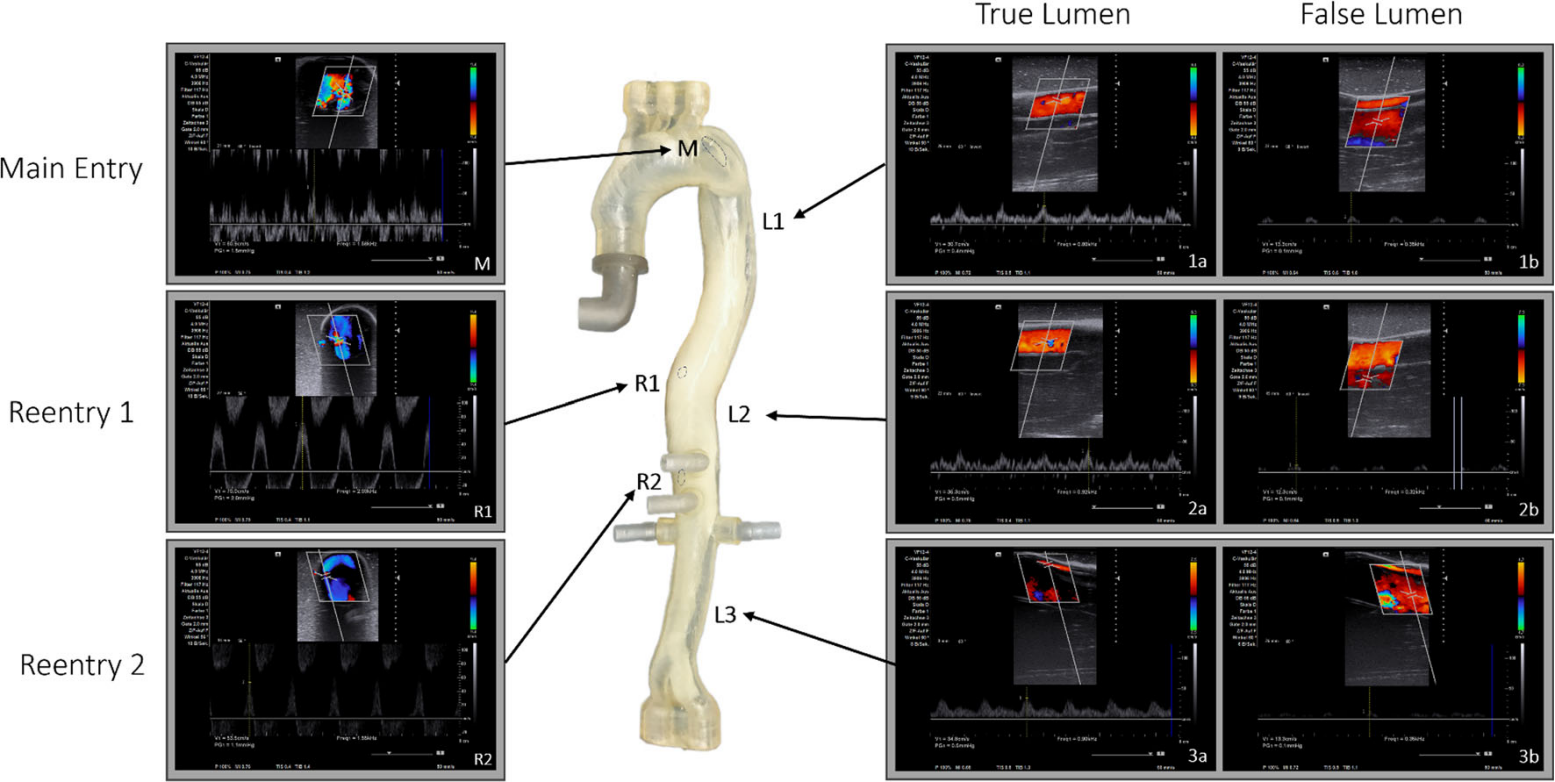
Ultrasound Velocity in true lumen (a) false lumen (b), main entry (M) and reentries (R1,R2)
